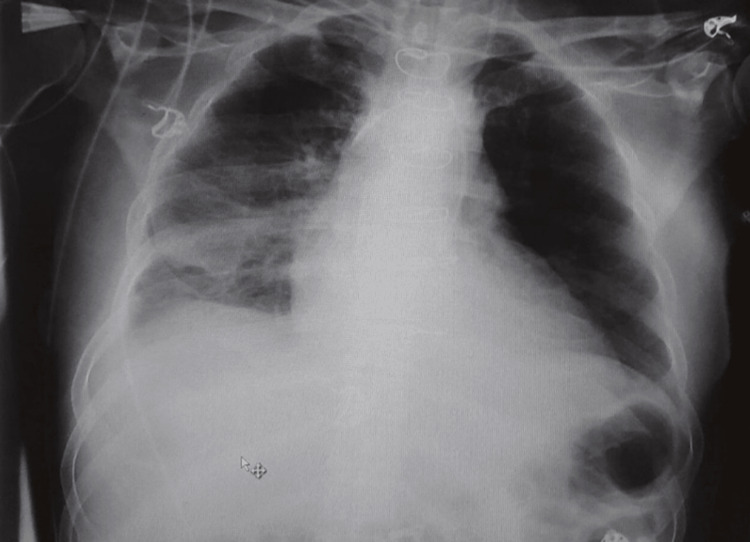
Chest X-ray of the patient on discharge showing lung infiltration on the right side; which improved compared to the previous ones
